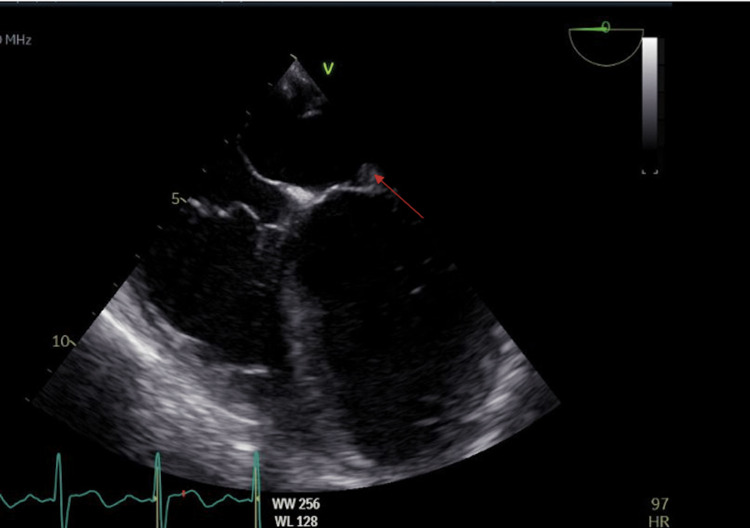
TEE image of the mitral valve vegetation in the four-chamber view. TEE, transesophageal echocardiogram
